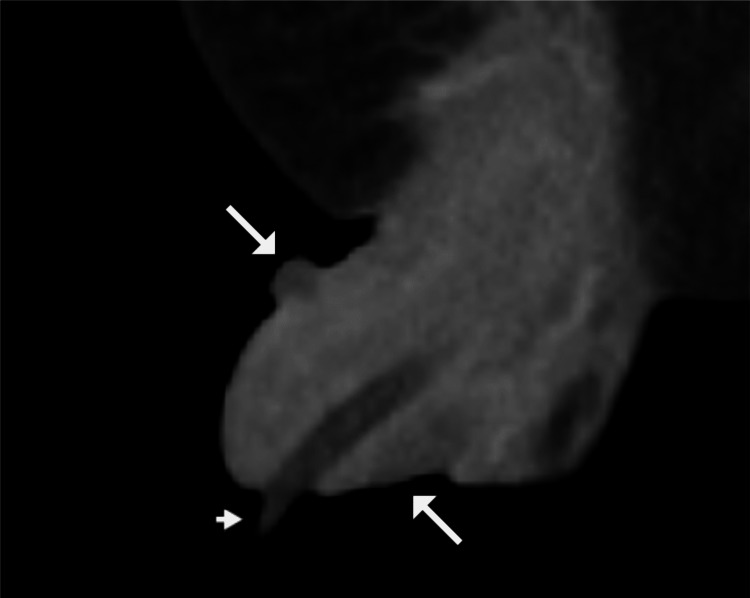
Coronal contrast-enhanced CT of the penis. Coronal contrast-enhanced CT of the penis shows a mildly thickened and hypoattenuating prepuce located proximal to the glans penis (long arrows) and a Foley catheter in the urethra (short arrow).
